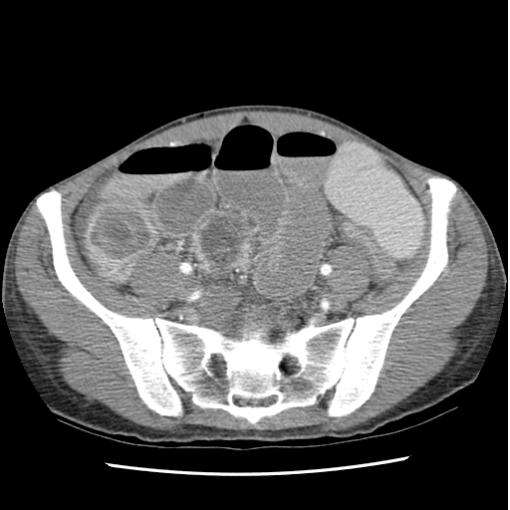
Computed tomography scan showing dilated loops of small bowel consistent with a possible small bowel obstruction with possible target sign in right lower quadrant raising the possibility of intussusception.
